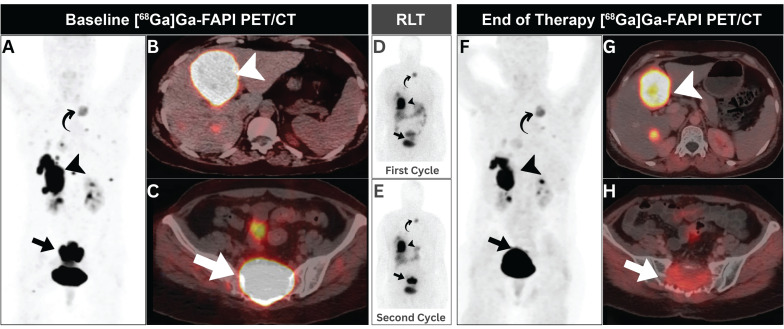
A 49-year-old woman with metastatic solitary fibrous tumor. (A-C) Initial [^68^Ga]Ga-FAPI PET/CT revealed intense uptake in hepatic (arrowheads), and sacral (arrows) metastatic lesions with minimal expression in other metastatic lesions elsewhere (curved arrow). (D, E) Post-treatment scintigraphy demonstrated satisfactory [^177^Lu]Lu-FAPI localization without side effects. (F-H) Follow-up [^68^Ga]Ga-FAPI PET/CT showed regression of sacral and hepatic lesions (arrows), with otherwise stable previous small metastatic lesions elsewhere (curved arrow). Adapted with permission from reference 38, copyright: © 2023 Indian Journal of Nuclear Medicine. Published under creative commons (License: CC BY-NC-SA 4.0).
